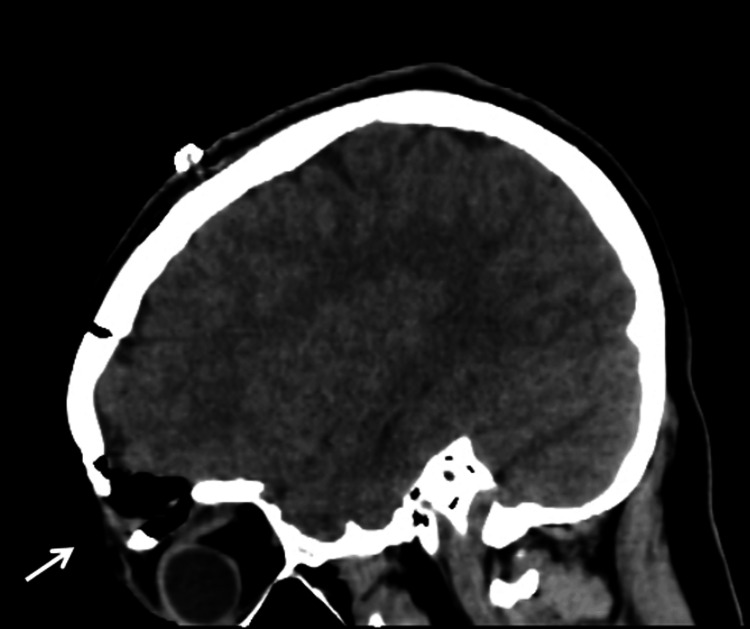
CT scan of the brain without contrast, post-resection of the lesion demonstrates expected post-operative changes (arrow) without immediate complication
